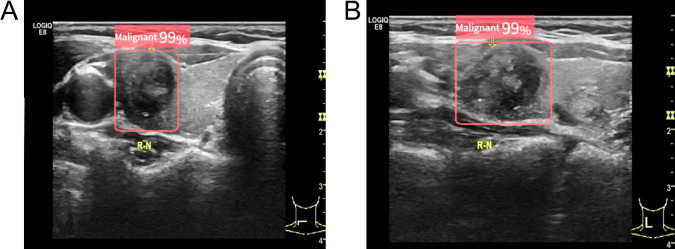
Representative case of concordant malignant AI output in both imaging planes. (A) Transverse-plane and (B) longitudinal-plane ultrasound images of a thyroid nodule in a 27-year-old woman show concordant high malignant-risk output (99%) on AI analysis. The final AI classification was “Possible Malignant.” Postoperative pathology confirmed papillary thyroid carcinoma.
